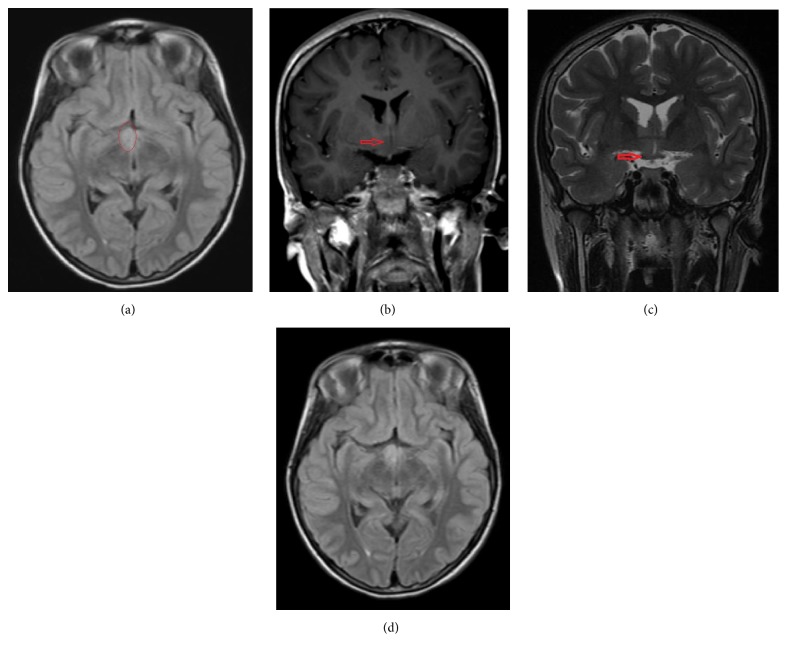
MRI of the brain. (a) Axial FLAIR image demonstrates asymmetric enlargement with abnormal FLAIR hyperintense signal within the right hypothalamus (red circle). (b) Coronal postcontrast T1 weighted image demonstrates no abnormal enhancement within the enlarged right hypothalamus (red arrow). (c) Coronal T2 weighted image demonstrates the mass abutting the lateral margin of the third ventricle and the superior margin of the optic chiasm (red arrow). (d) Follow-up axial FLAIR image, approximately 3 years later, demonstrates no significant change in size and abnormal hyperintense signal within the lesion.
